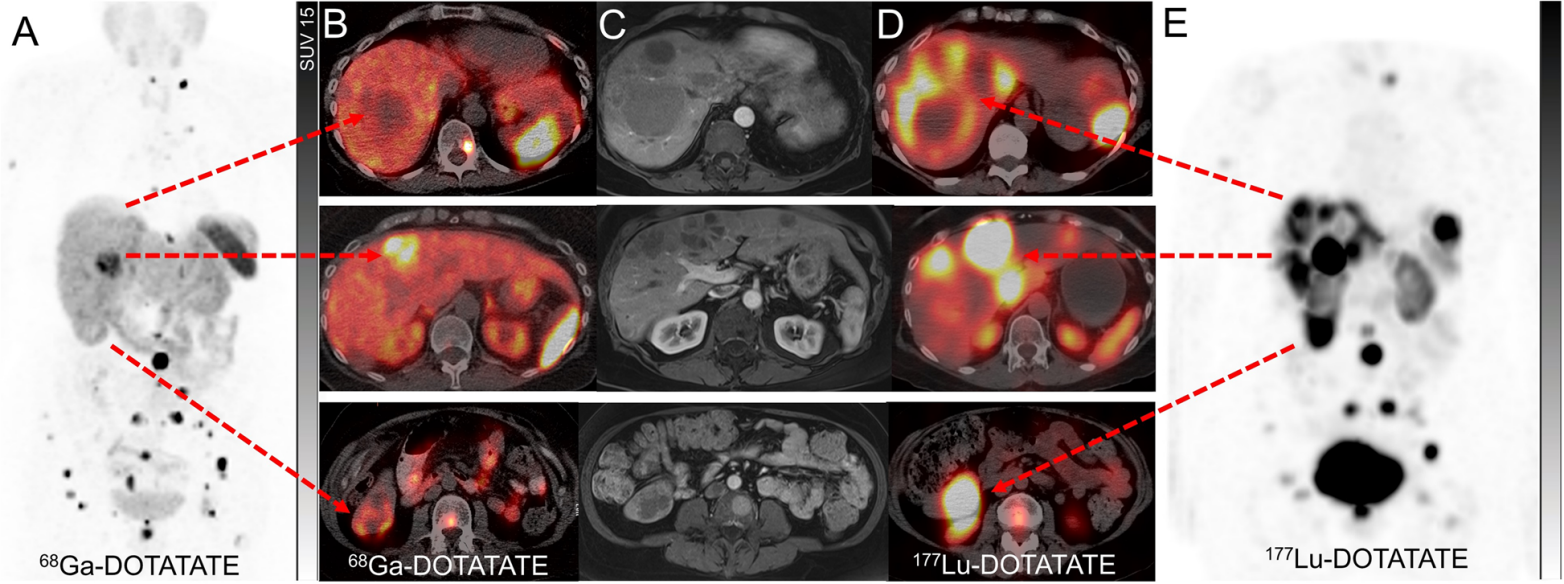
A patient with rectal NET (G2, Ki 67 12%) with progressive disease despite long-acting somatostatin analogues underwent ^68^Ga-DOTATATE PET (A), which showed intense uptake in the mesorectal lymph nodes and bone lesions but sufficient uptake only in one of the hepatic metastases (Krenning score 3–4) on PET/CT (B), while other contrast-enhancing lesions on MRI (arterial phase, C) had uptake similar to background liver (Krenning score 2). Contemporaneous ^18^F-FDG PET did not show any avid disease (not shown). Following discussion at the NET multidisciplinary team meeting, the decision was made to proceed with ^177^Lu-DOTATATE but only proceed with further cycles if high tracer retention is noted on post-treatment SPECT imaging. Post-treatment ^177^Lu-DOTATATE SPECT/CT (D) and SPECT (E) imaging showed high tracer retention (Krenning score 4) in all hepatic lesions, including lesions that showed low ^68^Ga-DOTATATE. The patient proceeded with 4 cycles of ^177^Lu-DOTATATE
